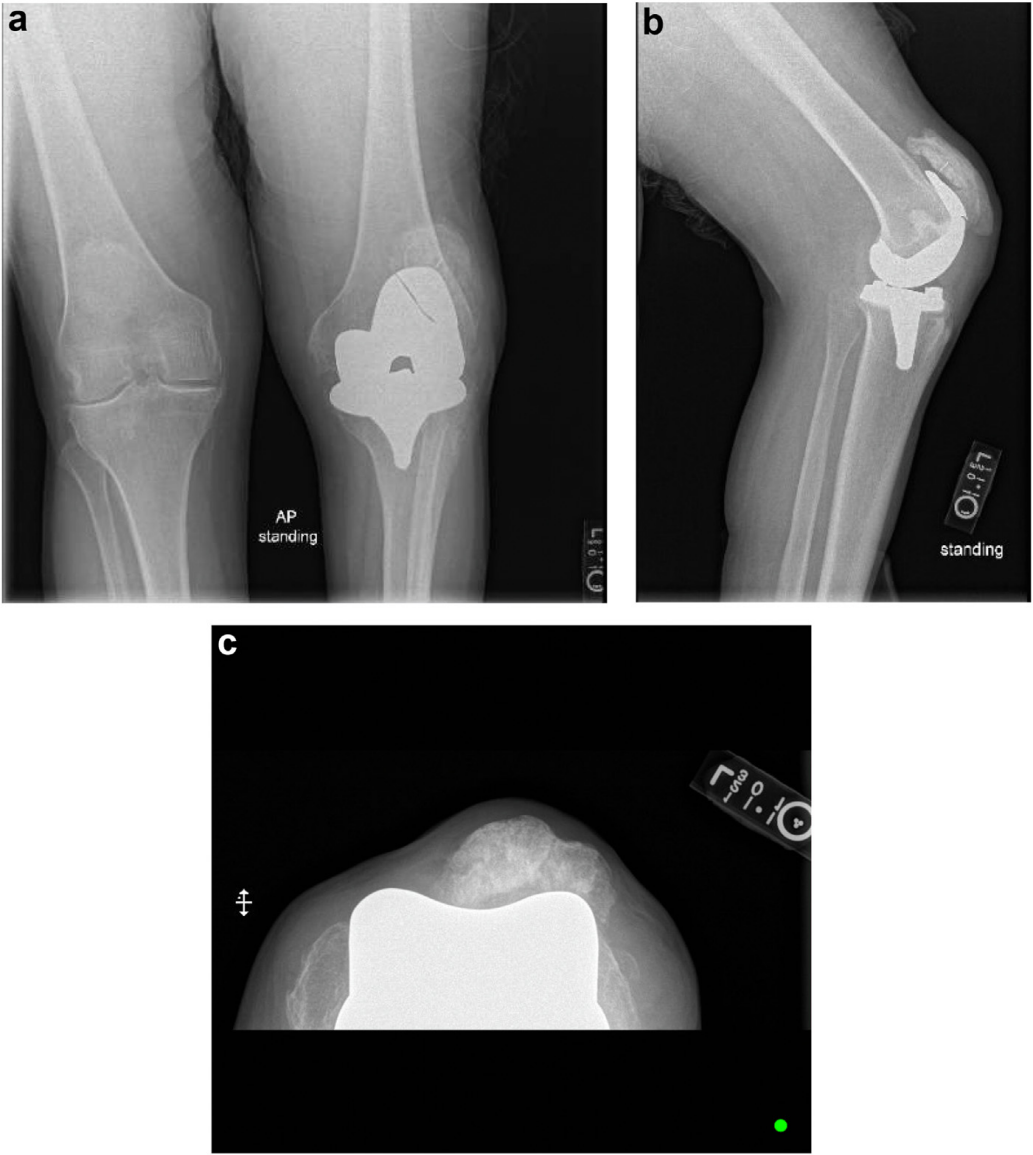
(a) Anteroposterior, (b) lateral, and (c) sunrise radiographs of left knee on presentation 12 years after primary TKA.
